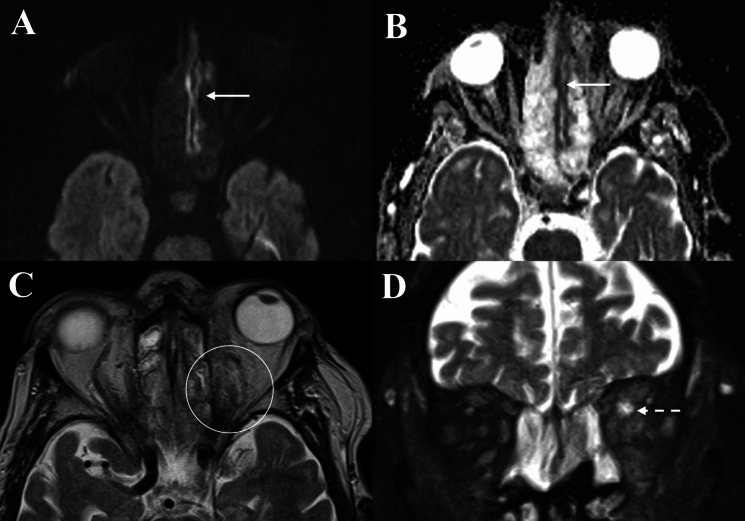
Orbital imaging of a 75-year-old male with invasive mucormycosis involving the left orbit. A and B Demonstrate regions of diffusion restriction along the mucosa lining of the ethmoid sinus on DWI, with low signal on ADC map (Solid arrows). C depicts an infiltrative mass within the extra- and intra-conal orbit fat on axial T2 (Solid circle). D T2 FS coronal images demonstrating loss of flow void and asymmetric enlargement of the left SOV consistent with superior ophthalmic vein thrombosis (Dashed arrow)
